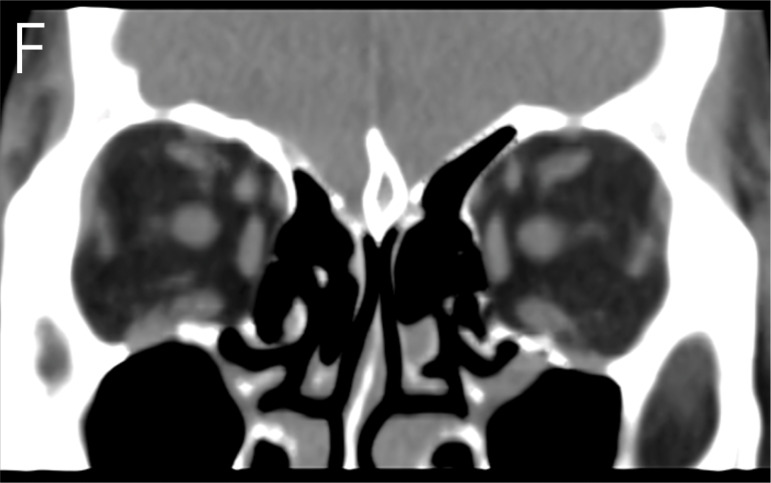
A 58‑year‑old male patient presented with bilateral eye protrusion for six months, blurred vision in the right eye for 15 days, and restricted eye movement. Axial (A, B), Coronal (C) post‑contrast T1 fat‑saturated MRI and axial (D) CT showed tortuous and thickened periorbital nerve tissue (arrows). A axial (E) and coronal (F) orbital CT examination 4 months after hormone therapy showed that the lesion improved significantly.
